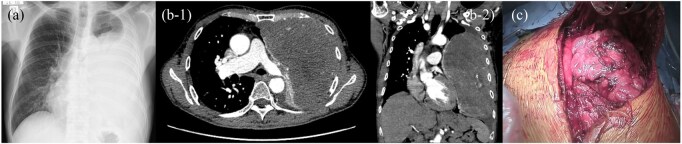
Preoperative Imaging and Intraoperative Findings. (A) Chest radiograph showing a large left thoracic mass. (B-1 and B-2) Contrast-enhanced computed tomography (axial and coronal) showing a heterogeneous mass compressing the lung with near-total atelectasis. (C) Intraoperative view through a hemi-clamshell incision showing a giant tumour adherent to pleural surfaces.
